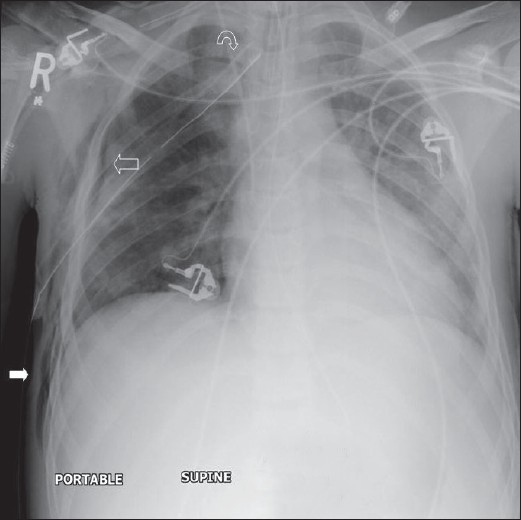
A portal supine chest radiograph showing surgical emphysema (solid white arrow) following placement of chest drain (curved arrow). Note the shallow pneumothorax (hollow white arrow). Surgical emphysema makes diagnosis of a pneumothorax difficult
